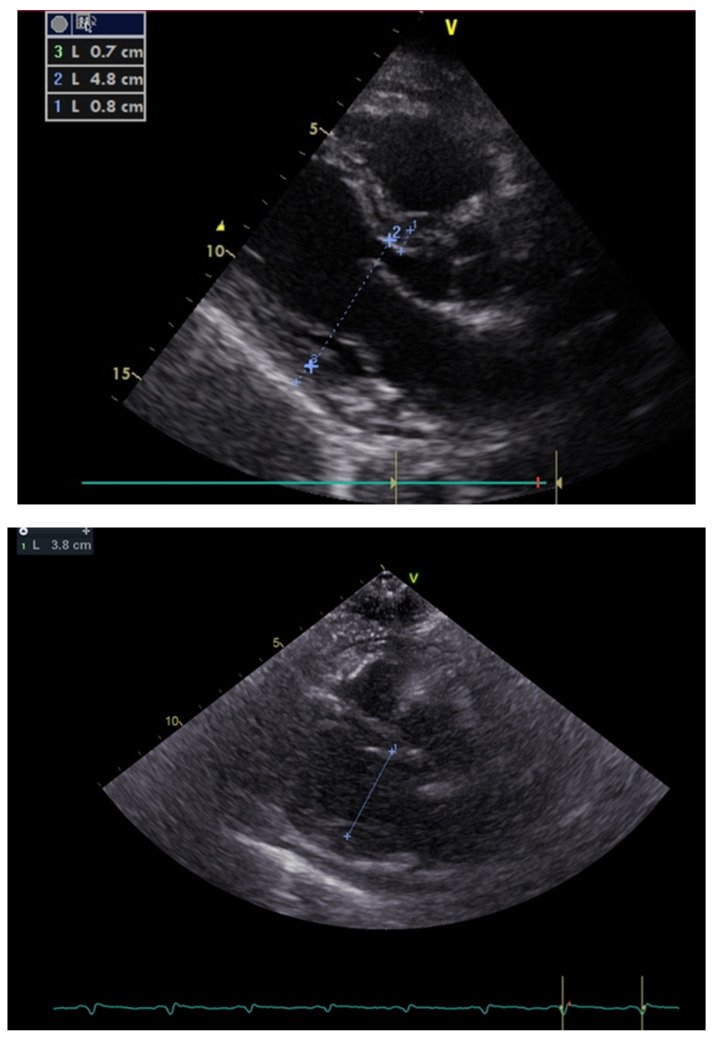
Markedly decreased left ventricular diastolic diameter from long axis parasternal view within 20 days.
